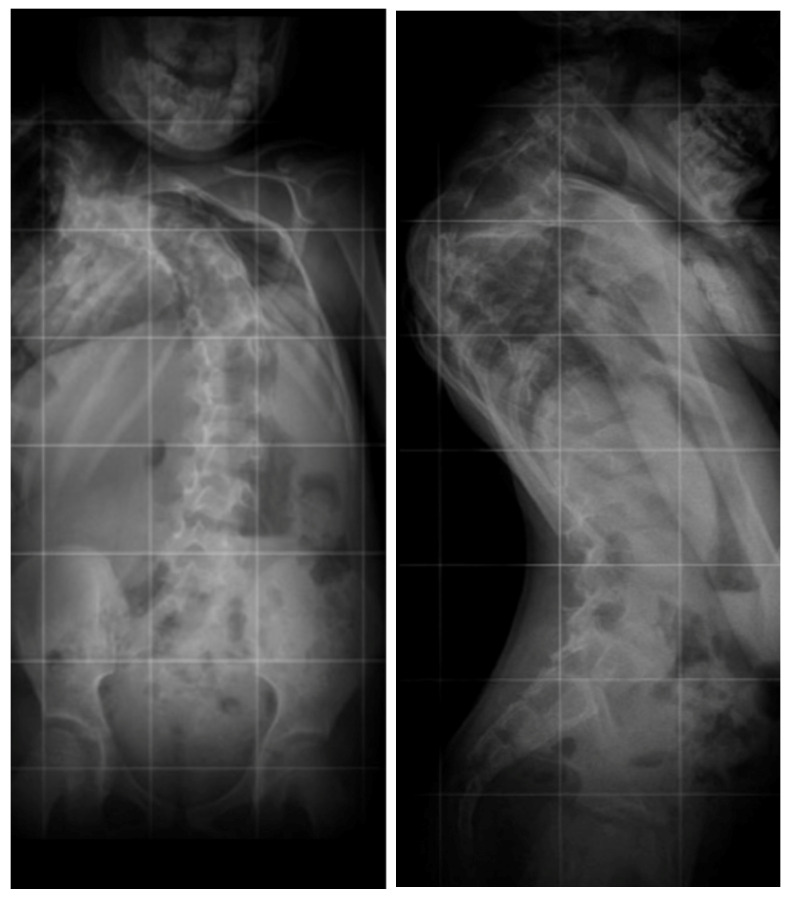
Standing X-rays of the five-year-old girl before surgical treatment.
